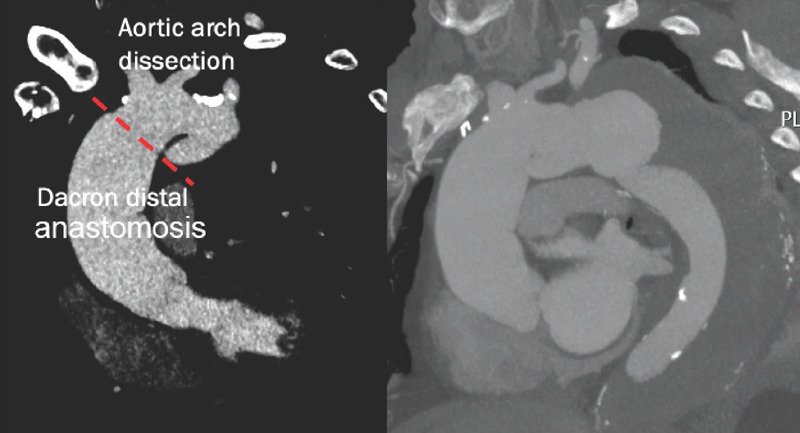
Preoperative computed tomography angiography showing a huge
